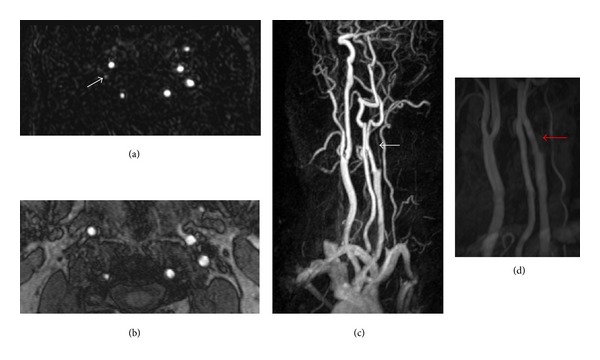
Forty-nine-year-old male patient with high-grade asymptomatic stenosis of the right external carotid artery detected with ultrasonography. While a filiform stenosis is seen on the contrast enhanced images (white arrow), the lumen of the right internal carotid artery is not seen on TOF images and the finding was interpreted as occlusion (red arrow). (a) Axial reformat of the subtracted contrast-enhanced MR angiography (CEMRA), (b) maximum intensity projection (MIP) of the CEMRA dataset, (c) axial time-of-flight (TOF) MR angiography image, and (d) MIP of the TOF MRA dataset. The much smaller axial field-of-view of the TOF MRA is clearly seen on the MIP images.
